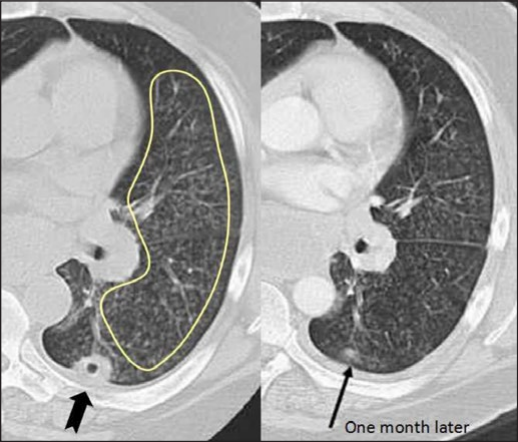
Rheumatic nodule.* A 46-year-old male with long-standing rheumatoid arthritis was immunosuppressed due to ongoing methotrexate treatment. CT was requested for chronic cough. The CT showed multiple centrilobular nodules in the left lung (inside the marked area), which were bronchiolitis due to his RA. In addition, a subplueral cavitary nodule is seen (thick arrow). A month later, the nodule was slowly dissipating (arrow).
